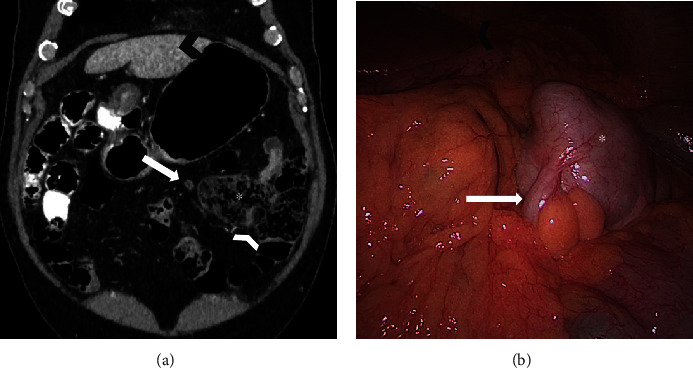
(a) Coronal reformatted contrast-enhanced CT image and (b) laparoscopic view demonstrate malrotation of the bowel with the ileocaecal valve (white arrowhead), the caecum (asterisk), and the normal appendix (white arrow) placed on the left side of the abdomen. Left hepatic lobe: black arrowhead.
